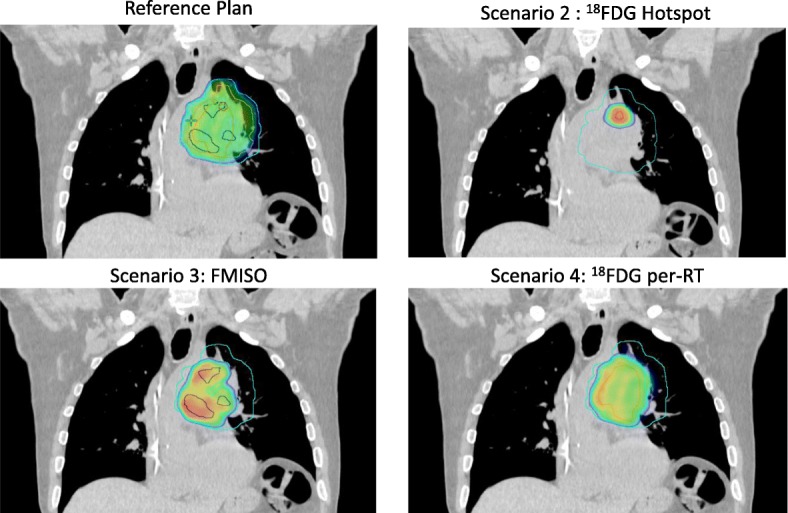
Example of dosimetry. Representation of dose (95% to maximum dosi) fr reference plan (66Gy) and three scenario of planification based on PET. PTV are blue, hotspot (BTVm1) is red, FDG pre-RT (BTVm2) is orange and FMISO (BTVh) is dark blue. For each plan the PTV 66 is represented
